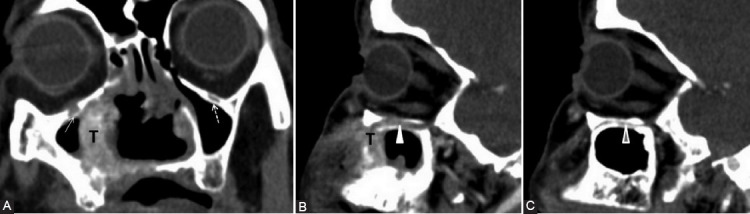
Nasal squamous cell carcinoma (T) with maxillary extension. Coronal post-contrast computed tomography image (A) shows enlargement and contrast enhancement of the right infraorbital nerve in its corresponding foramen (arrow). The enlarged infraorbital canal (white arrowhead) shown on the sagittal view (B, C) is a pathway for the PNS toward the inferior orbital fissure and then the pterygopalatine fossa. Notice the normal left infraorbital foramen fat pad (dashed arrow) and canal (gray arrowhead).
